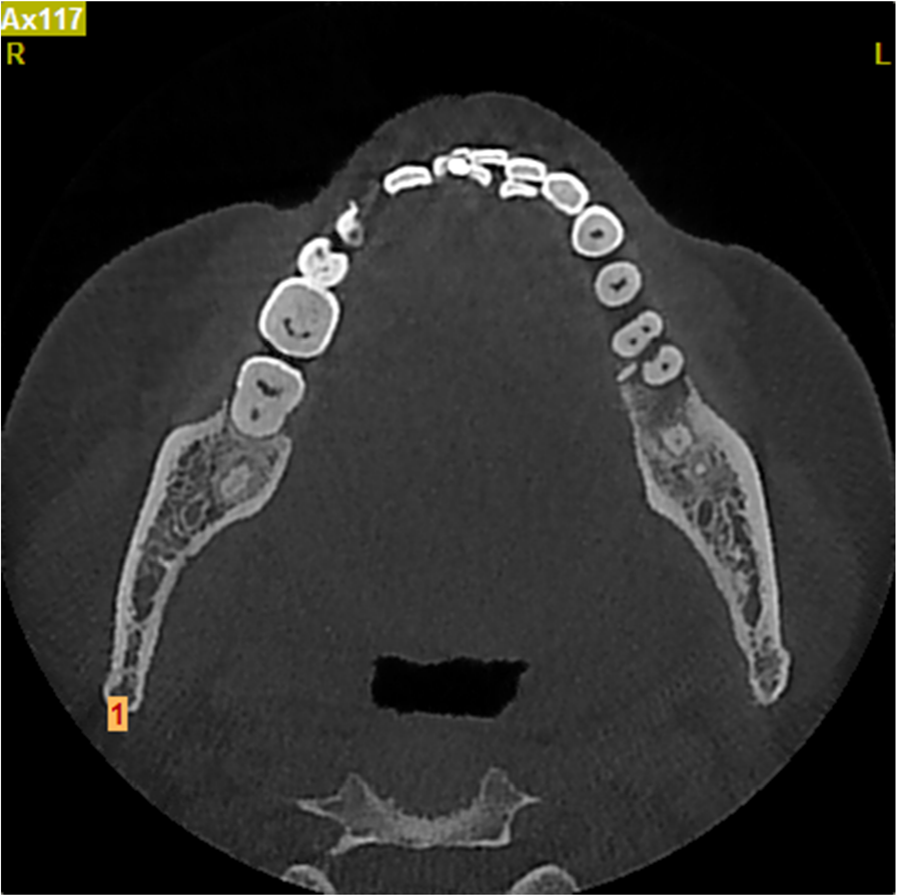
Computed tomography horizontal section view.
